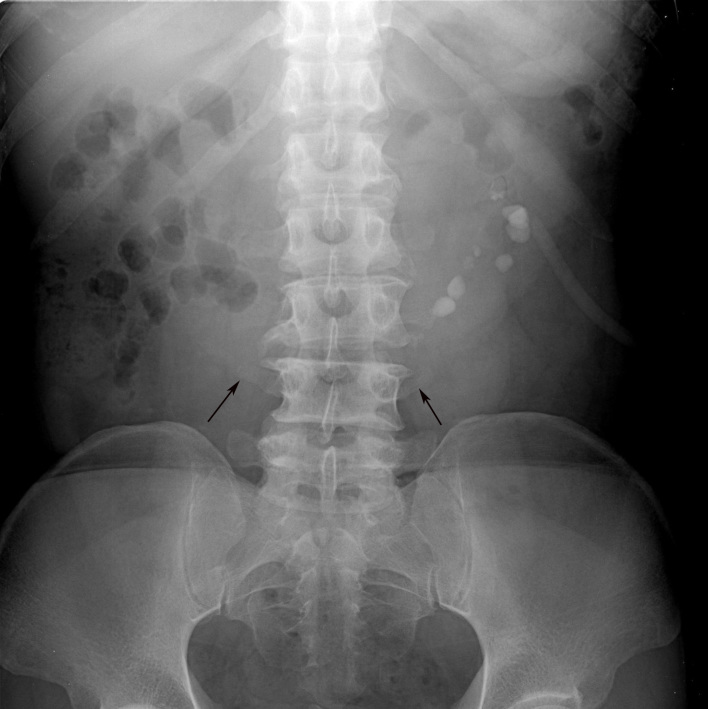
Abdominal radiograph in a 50-year-old man who presented with abdominal pain shows medial deviation of the lower poles of both kidneys and the visible isthmus (arrows). There are multiple stones in the left kidney and stones in the lower pole locate medially while stones in the upper pole locate laterally.
